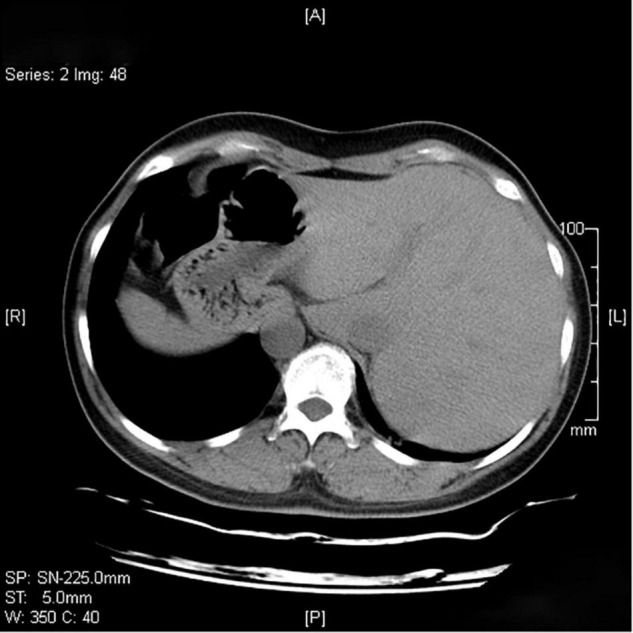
Computed tomography scan of the abdomen showing liver on the left.
